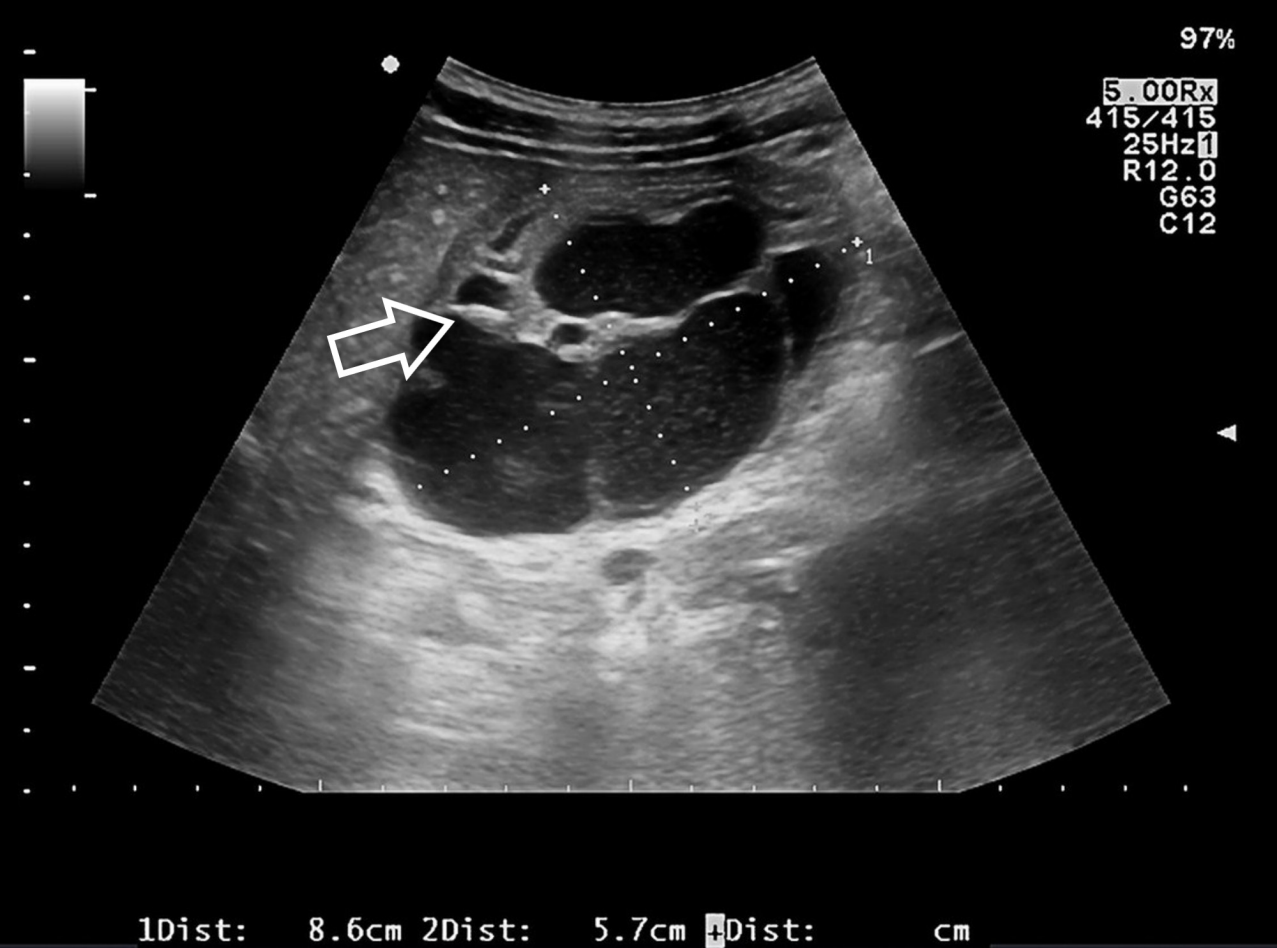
Endoscopic ultrasonography. Heterogeneous mass (8 x 6 cm) in the third and fourth layers of the gastric wall with fluid and a solid (arrow) components.
